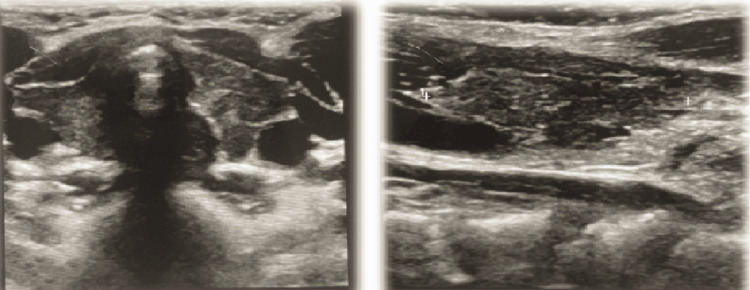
The neck ultrasound shows a mild form of chronic parenchymal changes of the thyroid gland in the second case.
